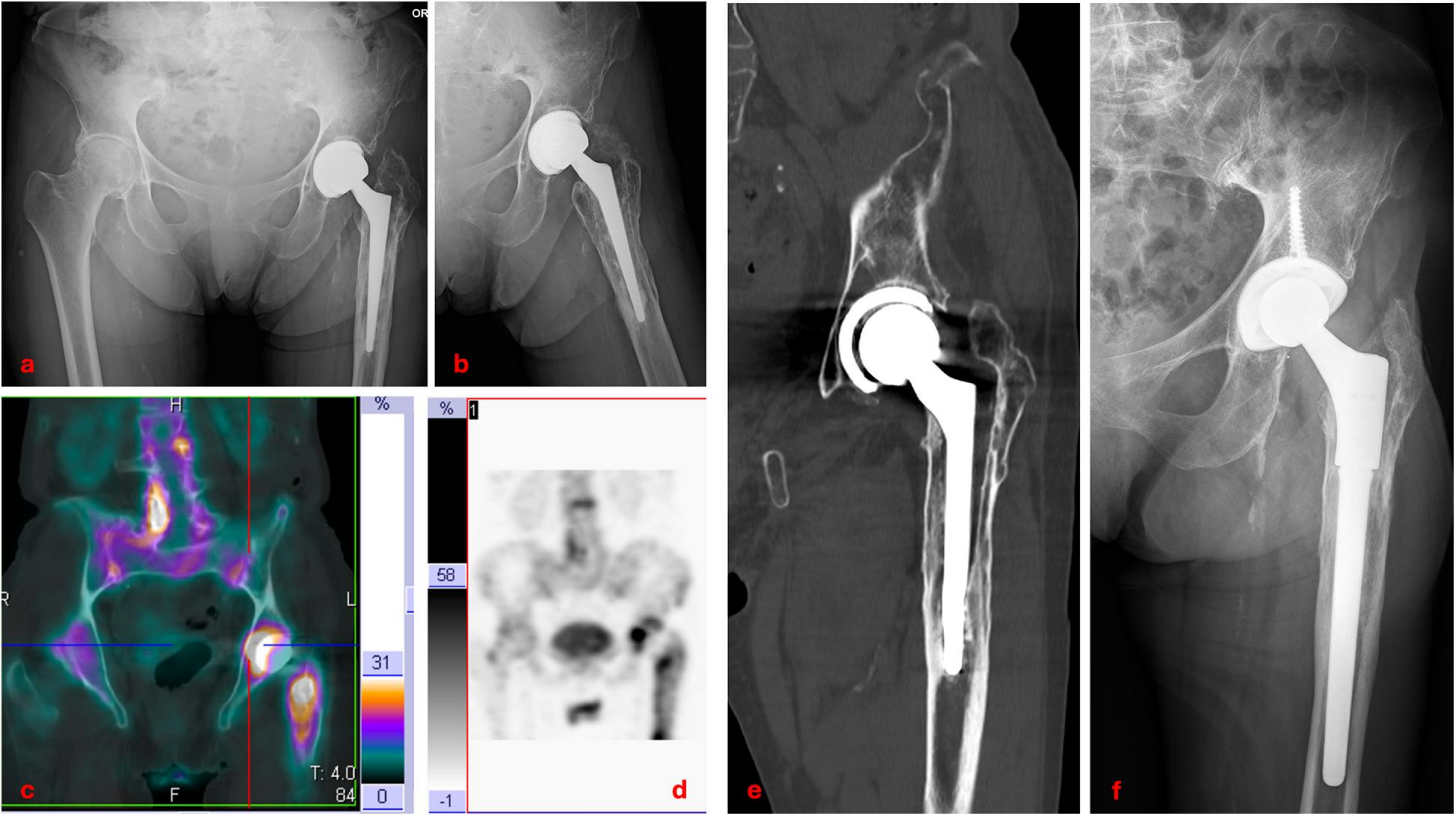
Clinical case of aseptic loosening of total hip arthroplasty: anteroposterior and lateral radiographs (a,b); baseline three-phase bone scintigraphy with 99mTc showing increased uptake around the prosthesis (c,d); coronal CT scan demonstrating loosening of both the femoral stem and acetabular cup (e); postoperative radiograph after revision surgery with a new modular stem (f).
